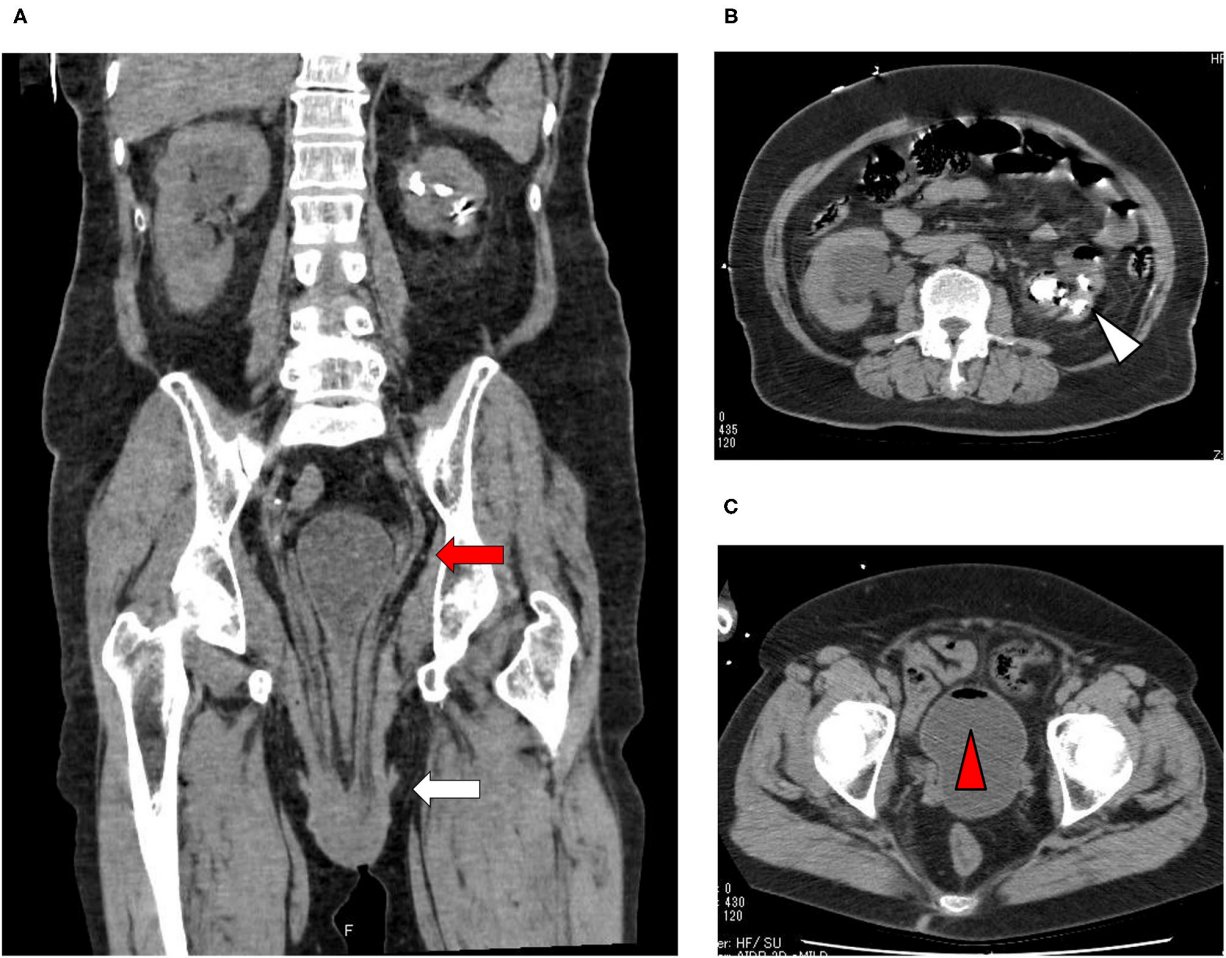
(A) Abdominal CT revealed renal calculus and hydronephrosis, and pelvic CT revealed lower shift of bladder (red arrow) and severe uterine prolapse (white arrow). (B,C) Abdominal CT showed emphysematous pyelonephritis (white triangle), and pelvic CT showed emphysematous cystitis (red triangle).
